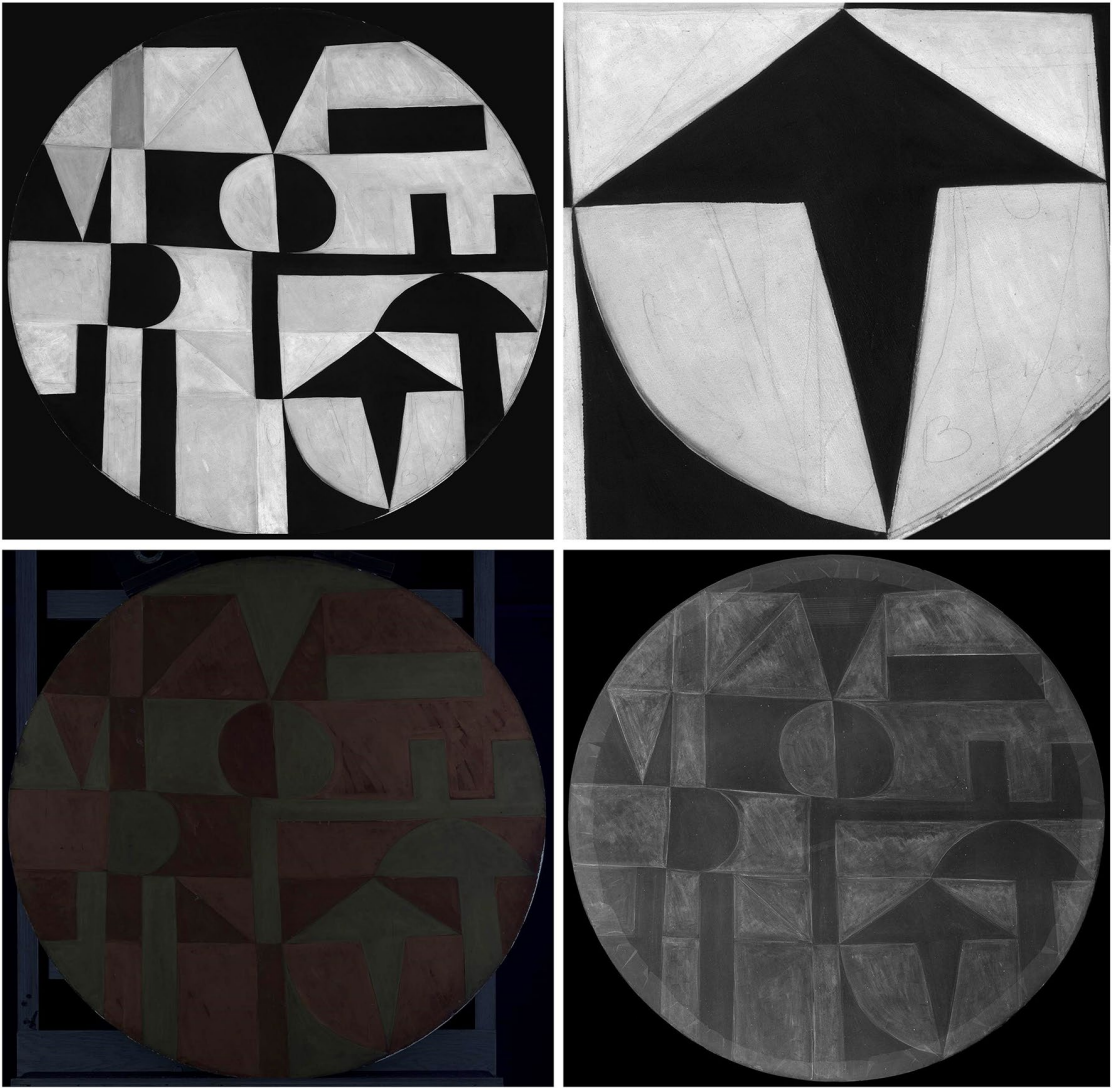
From top left to bottom right: IR reflectogram with detail, UV photograph, and X-radiograph of *Iberic* (1949)
